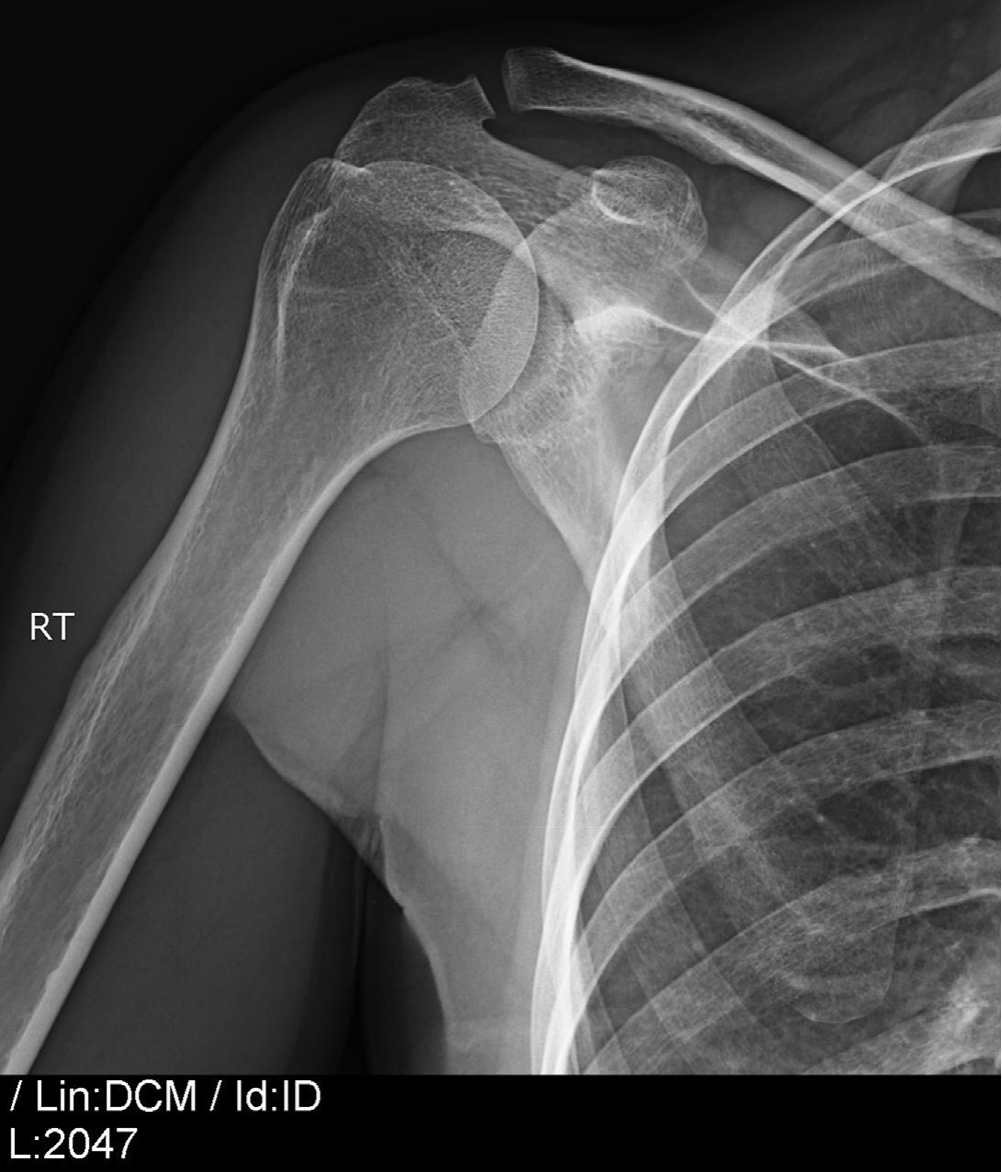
X-ray of the shoulder of the previous patient after the end of the treatment protocol 25 days after the drilling of the deposit.
